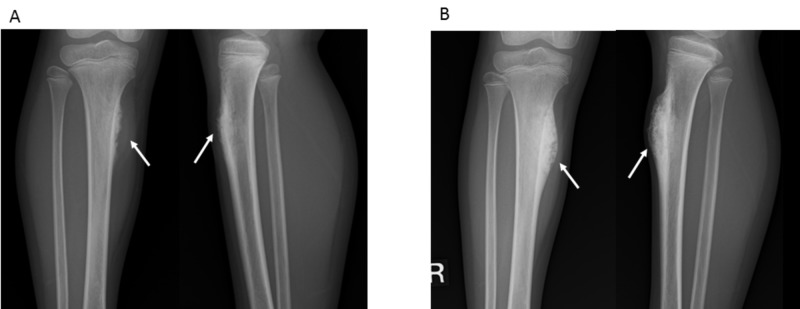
Radiograph of tibial periosteal osteosarcoma before and after neoadjuvant chemotherapy (NAC) A) Pre-neoadjuvant chemotherapy (NAC) anteroposterior and lateral radiograph of the lower leg show cortical thickening that is eroded by a broad-based soft tissue mass without involvement of the underlying cortex; B) Post-NAC anteroposterior and lateral radiograph of the lower leg show cortical thickening that is eroded by a broad-based soft tissue mass with intense calcification.
